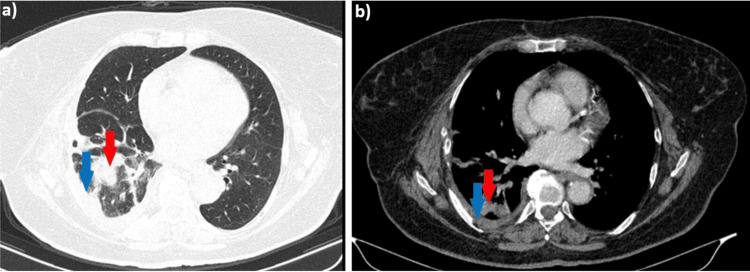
Contrast chest CT scan, (a) lung window, axial plane; (b) mediastinal window, axial plane, showing a nodular pulmonary lesion and pleural effusion
